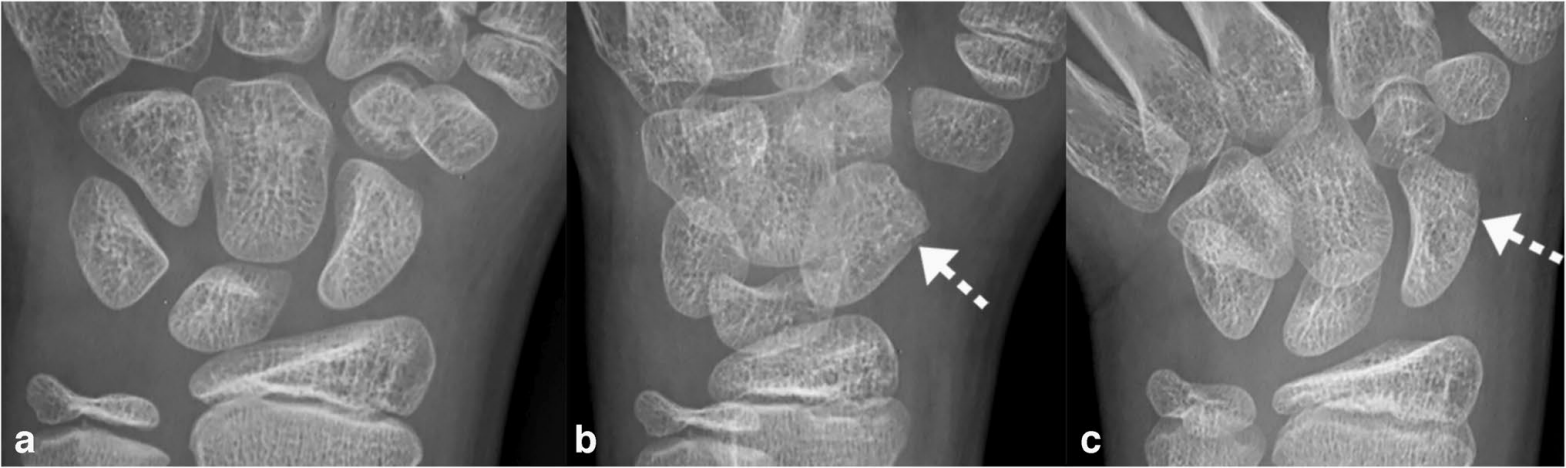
Distal pole fracture in a skeletally immature 11-year-old boy. PA (a), oblique (b), and PA scaphoid (c) radiographic views show a non-displaced fracture (dashed arrows) without articular involvement or perifracture findings. The patient was successfully treated with conservative treatment
